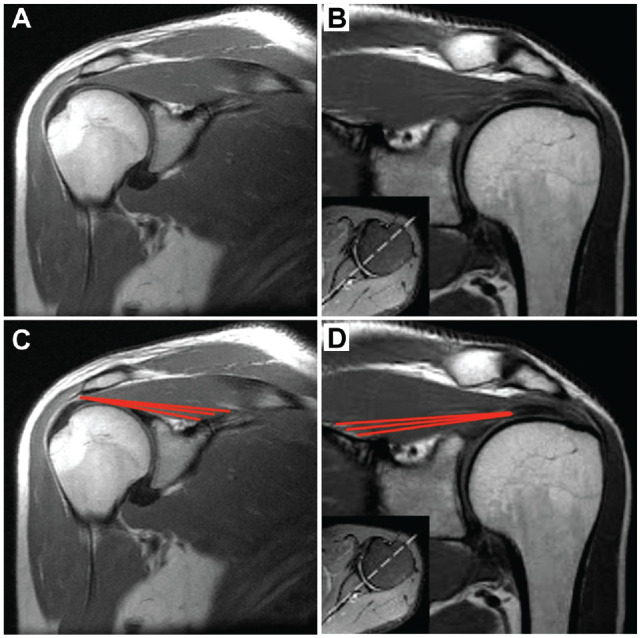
T1-weighted coronal magnetic resonance imaging (MRI) of the supraspinatus muscle. (A, B) Coronal oblique T1-weighted MRI section through the midbelly of the supraspinatus in 2 different patients. (C, D) Red lines indicate the approximate origin and insertion of the deep supraspinatus muscle fibers.
